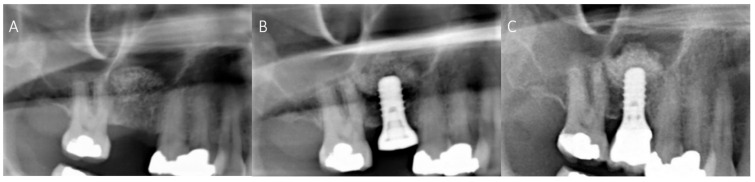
Radiographs of the right partial edentulous maxilla after maxillary sinus floor elevation (A), after dental implant placement (B) and at 2-year follow-up (C). Apical to the dental implant the graft material has been lifted upwards, resulting in a higher local tissue height at the implant position.
